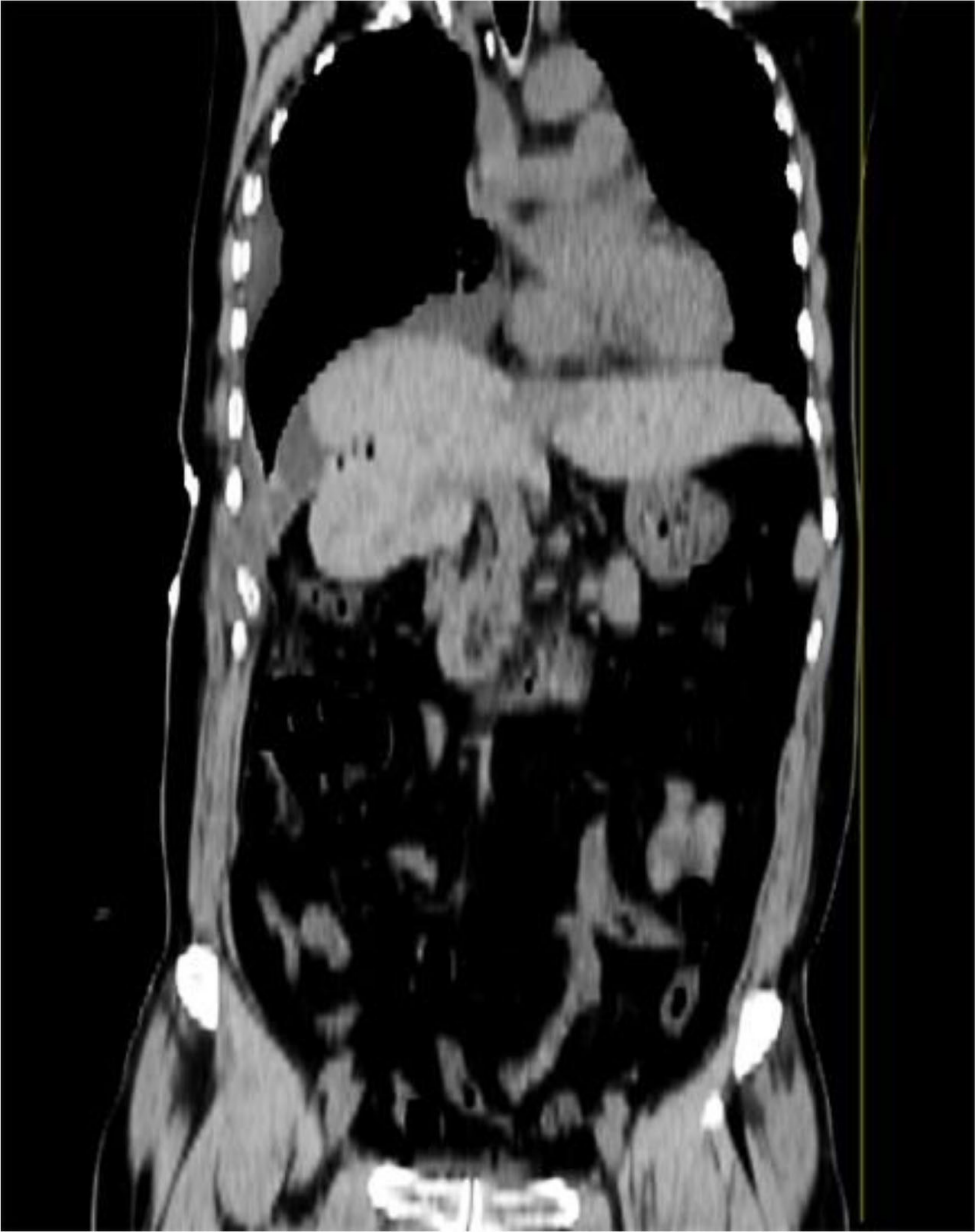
Representative images of abdominal CT after 10 days. Coronal CT showed that there was a small amount of fluid in the pleural cavity and that the liver had been repositioned into the abdominal cavity.
